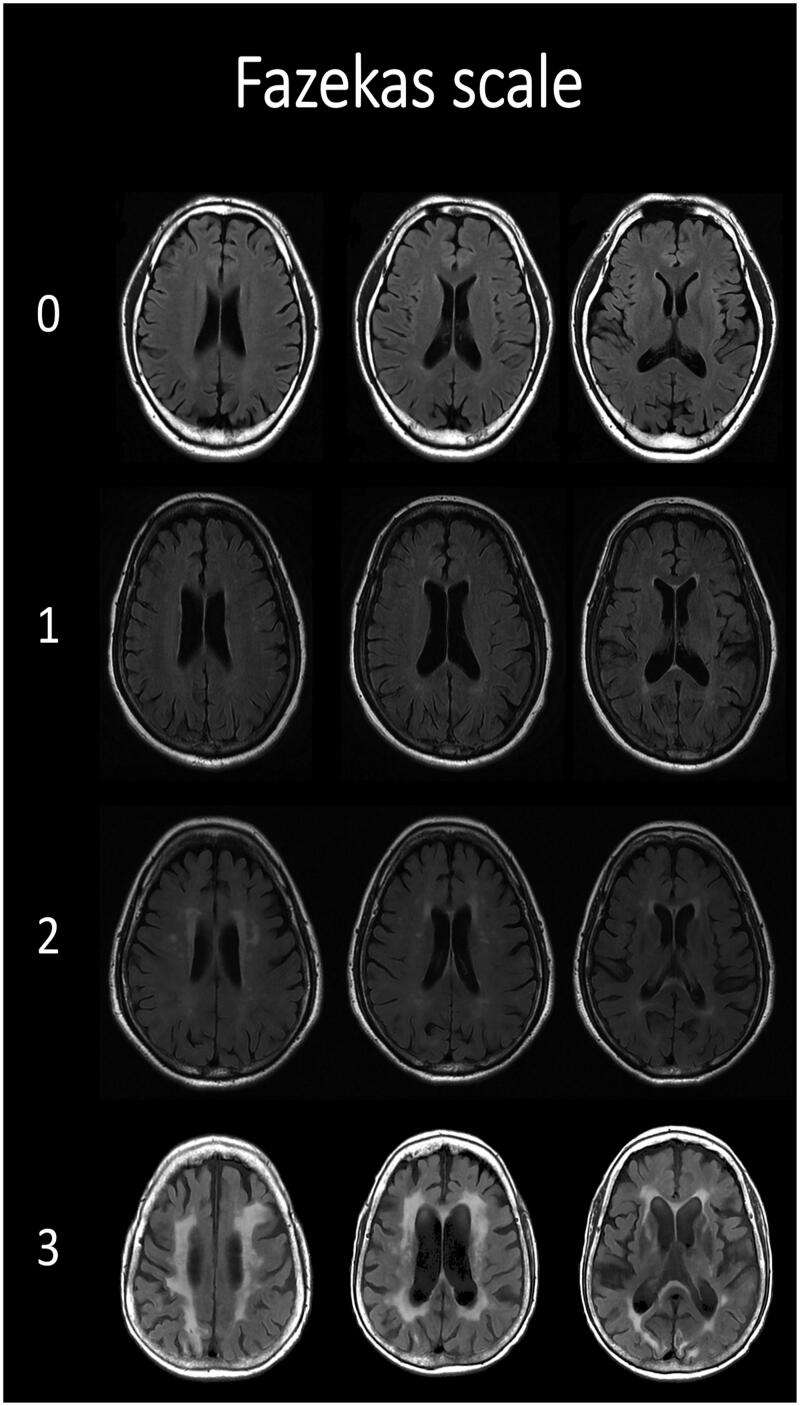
Fazekas scale of periventricular white matter hyperintensities in MRI examples.
